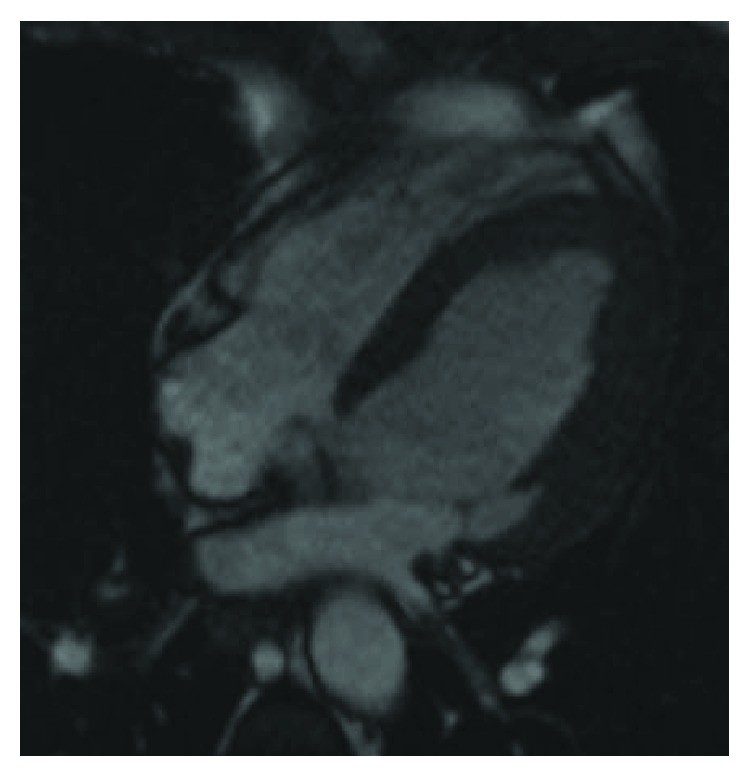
Cardiac magnetic resonance imaging 4-chamber view (still frame from a steady-state free precession (SSFP) cine sequence) taken 3 weeks after the previous study and on treatment (apixaban and antibiotics) demonstrating resolution of the apical mass.
